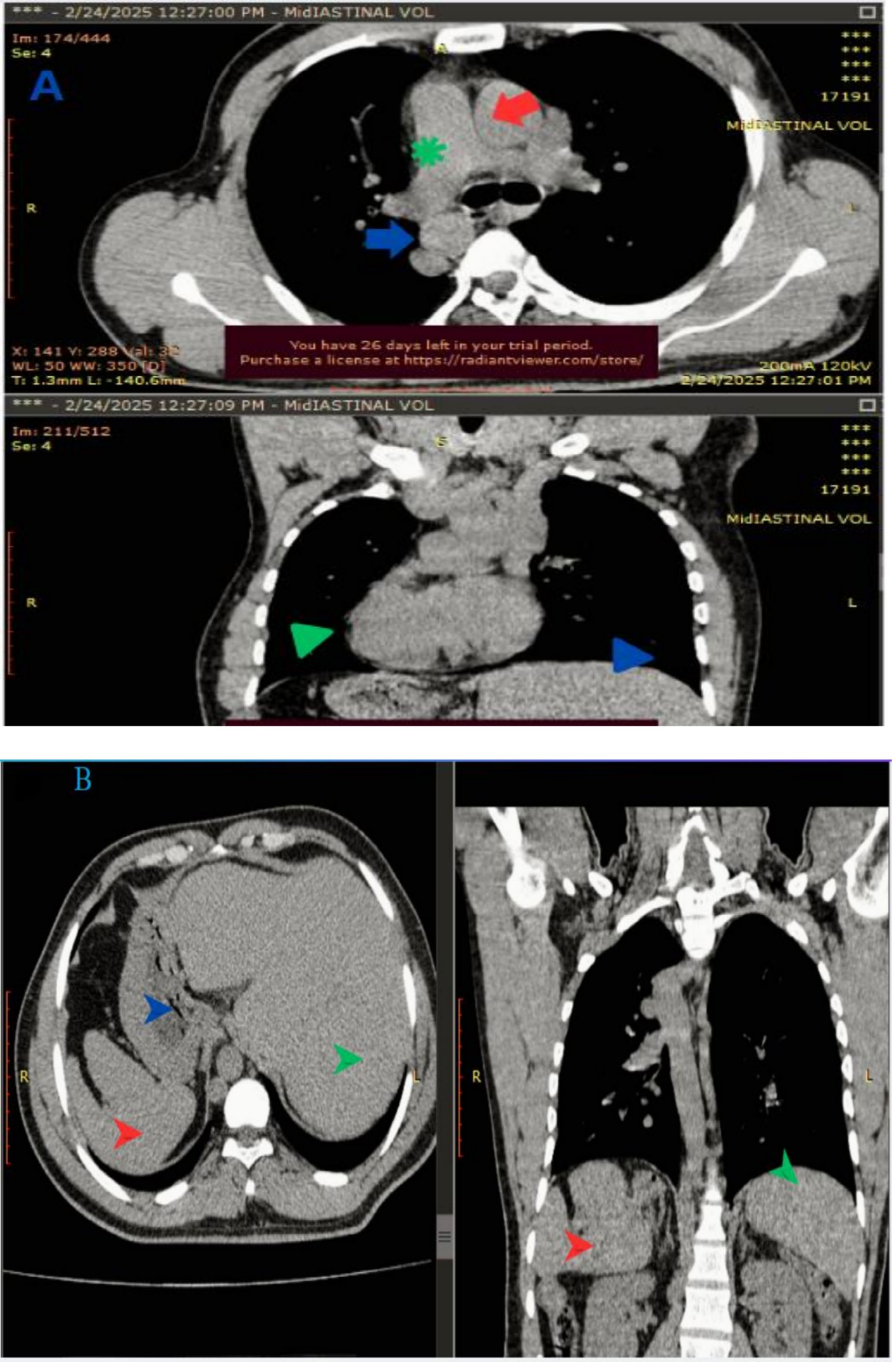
CT scan of the chest shows: (A) Pulmonary trunk (green asterisk) to the right, ascending aorta (red arrow) to the left and descending aorta to the right (blue arrow), the apex of the heart pointing to the right (green arrowhead). (B) Spleen (red arrow head), stomach (blue arrow head) and aorta visualized on the right side and liver (green arrow head) the left side.
